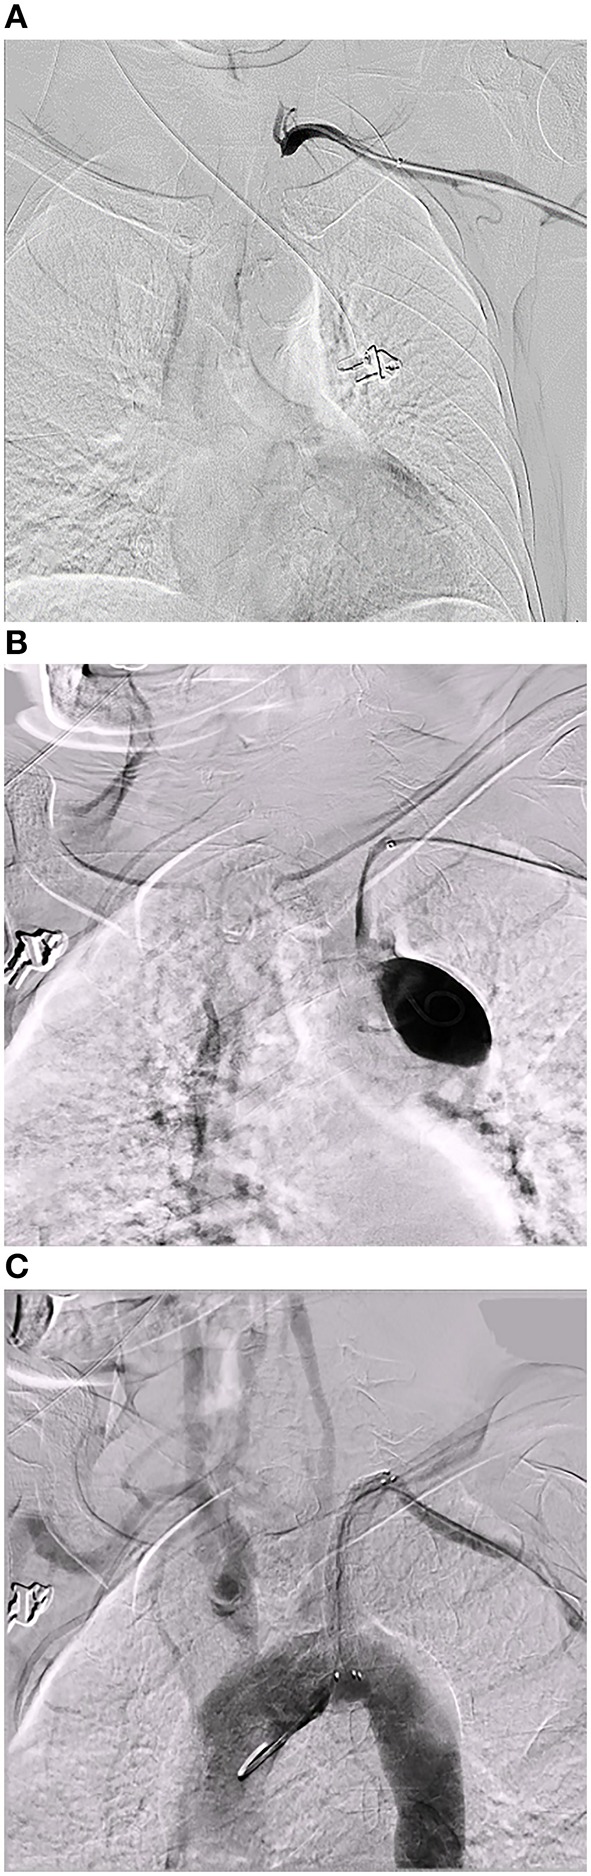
An arterial dissection. A 70 year-old male patient who presented with vertigo and left arm claudication for 2 years. CTA of the lower extremity showed bilateral iliac artery occlusion. (A) Angiography revealed a long segment occlusion at the beginning of the left subclavian artery. (B) After passing a guidewire through the lesion via the retrograde approach through the left brachial artery, angiography revealed an arterial dissection from the proximal segment of the left subclavian artery to the aortic arch. (C) A covered stent was implanted to treat the dissection, but the blood flow of the left vertebral artery was affected.
